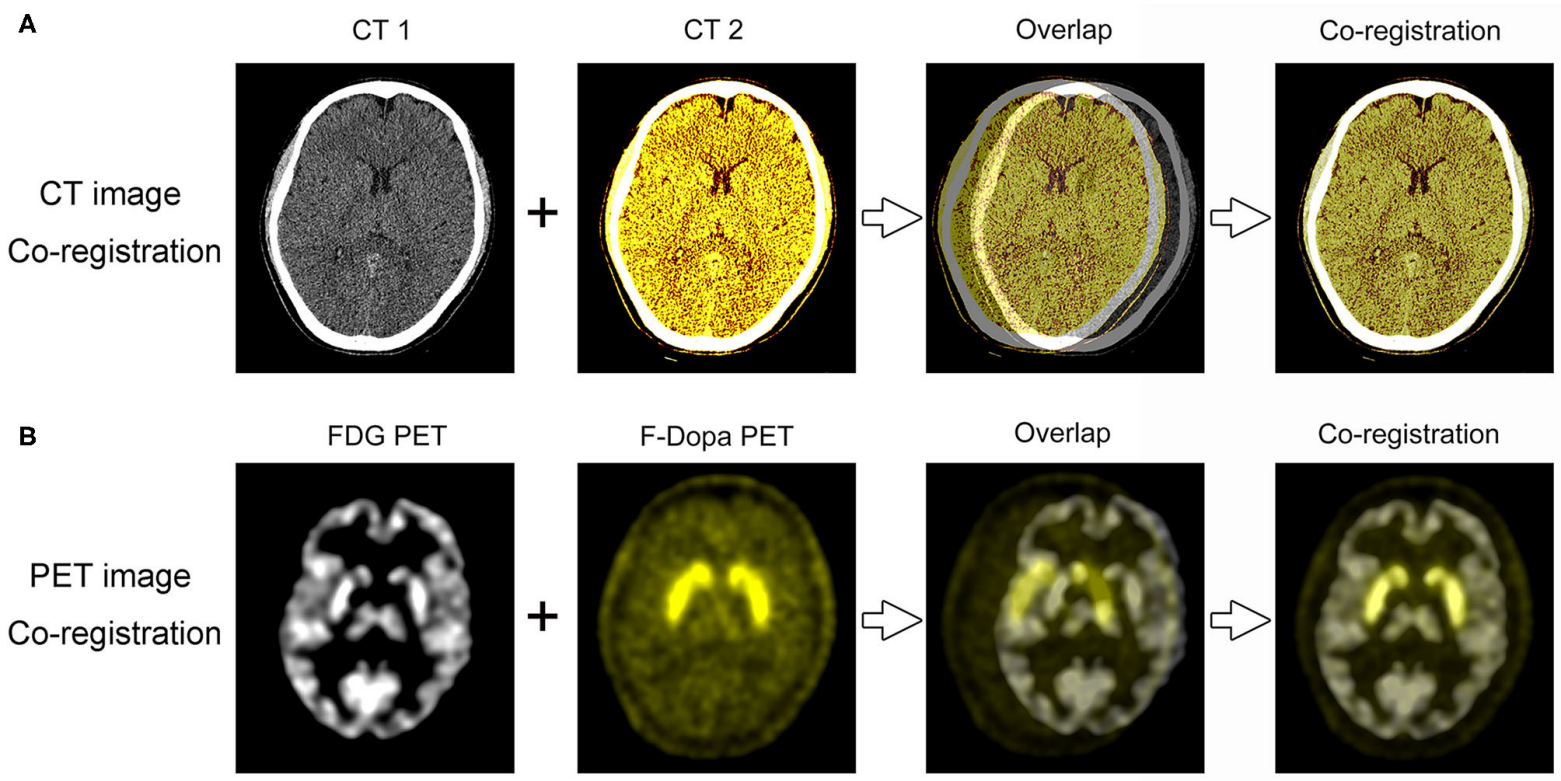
PET/CT image co-registration processing. (A) The first step was to make the co-registration of two CT images using 3D Slicer software. (B) The second step was to make automatic registration of the ^18^F-FDG and ^18^F-Dopa PET images.
